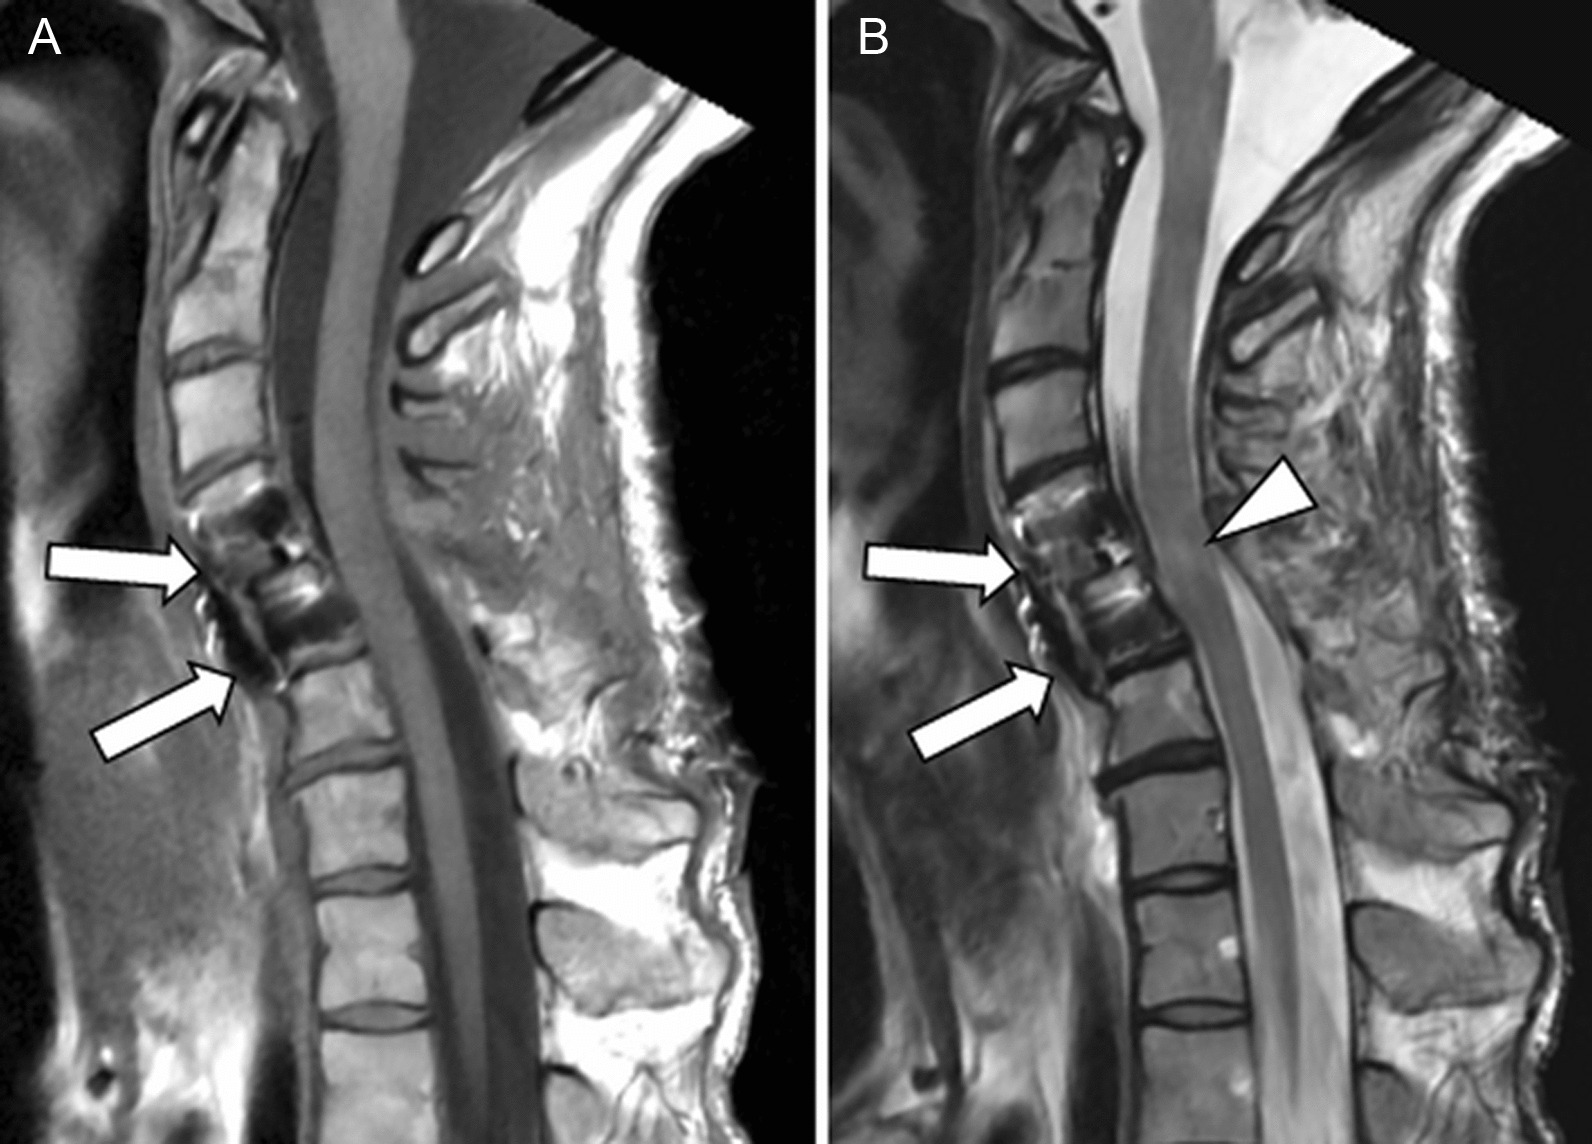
Postoperative magnetic resonance imaging: sagittal T1 (A) and T2 (B) weighted images showing realignment of the spine, return to normal dimensions of the spinal canal, and the presence of stabilization material (arrows). Note the presence of subtle residual myelopathy on the T2 image (arrowhead in B)
